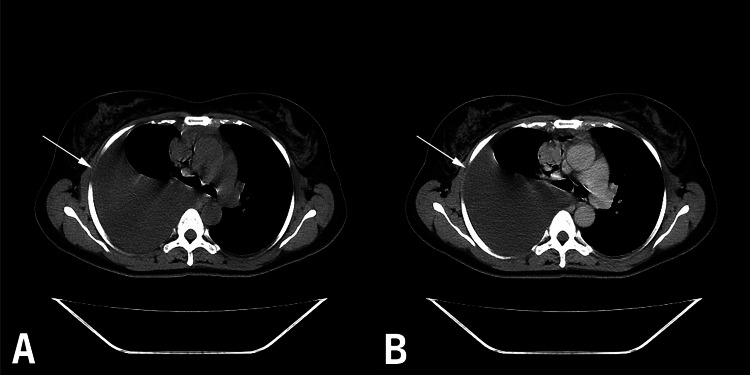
A. Plain CT (axial view) showing the pleural effusion on the right side; B. CT with contrast (axial view) showing the pleural effusion on the right side
